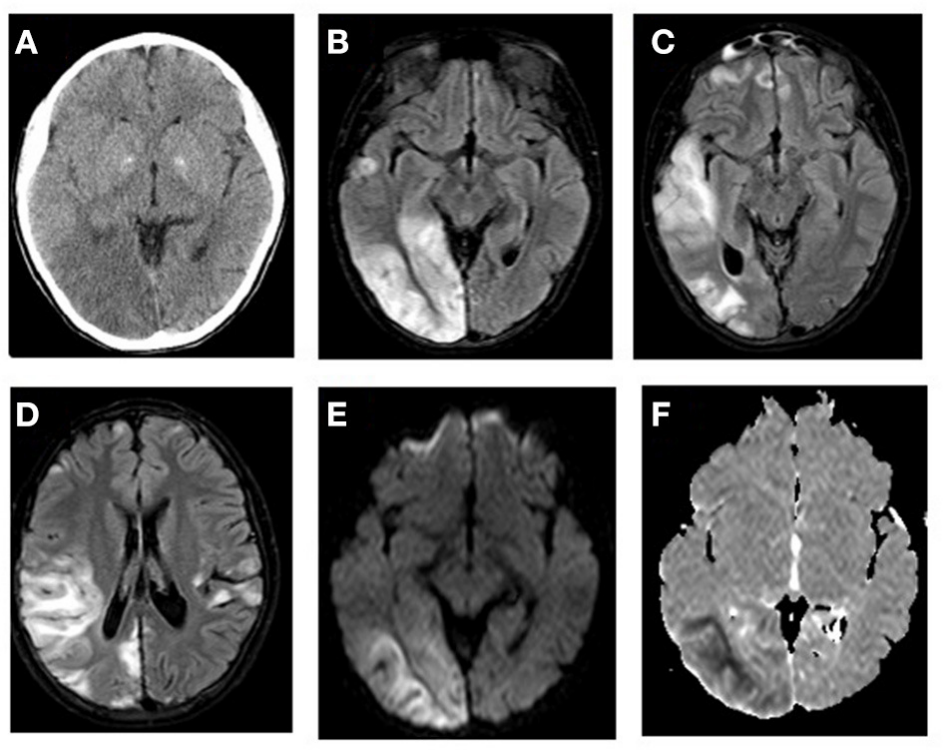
MTTL1*-related MELAS with stroke-like episodes. (A) CT scan shows hypodense areas in the right posterior temporal and occipital lobes with mass effect and effacement of the sulci on the surface of right cerebral hemisphere. FLAIR MR images (B–D) demonstrates bilateral multifocal cortical and subcortical white matter predominantly in the right temporo-occipital areas with swollen gyri. The lesions showed increased signal on diffusion weighted image (E) and low signal on ADC map (F) of the subcortical white matter related to the acute ischemic insult.
